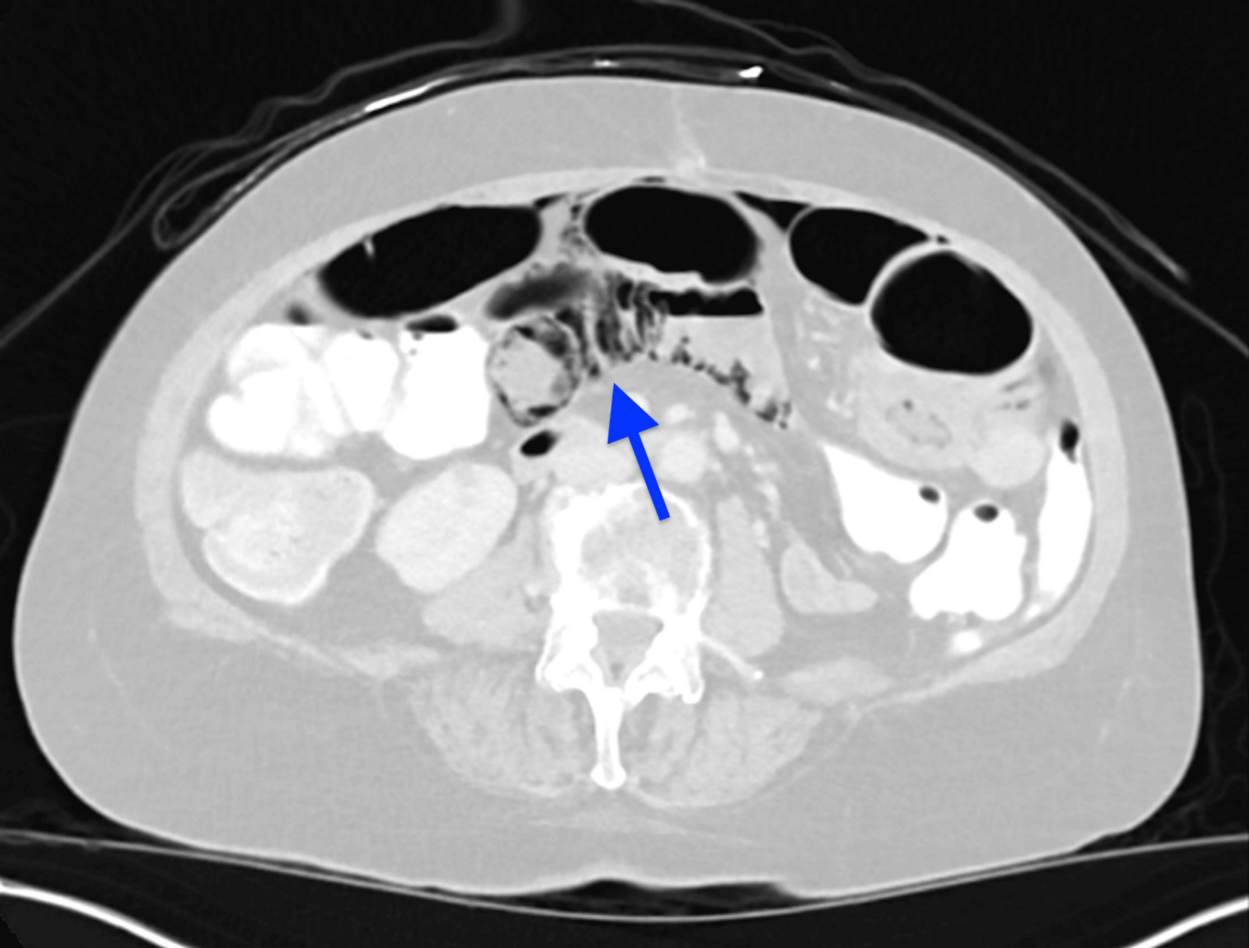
Representative image from the CT scan of the abdomen showing extensive small bowel pneumatosis (blue arrow)
